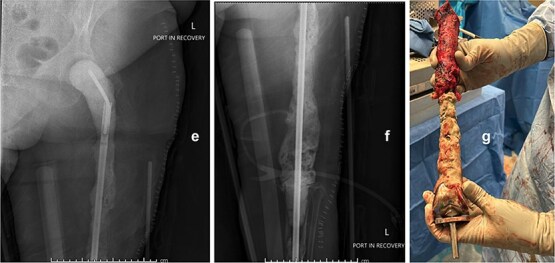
Intraoperative anteroposterior radiographs (e and f) after stage one, demonstrating a custom antibiotic-loaded total femur spacer constructed using an Ilizarov rod embedded in antibiotic cement and a fully cement-coated fusion nail extending from the acetabulum to the tibia. The resected right femoral implant is grossly visualized (g) prior to spacer placement.
